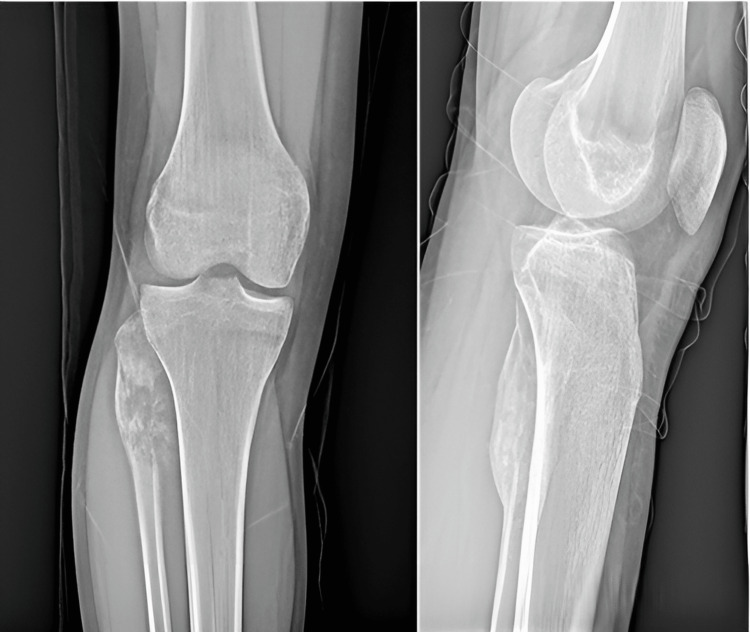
Direct radiograph of the patient who presented with complaints of pain and swelling in the right knee A lesion can be seen containing opacities and showing a periosteal reaction, which was destroying the cortex in the proximal fibula.
